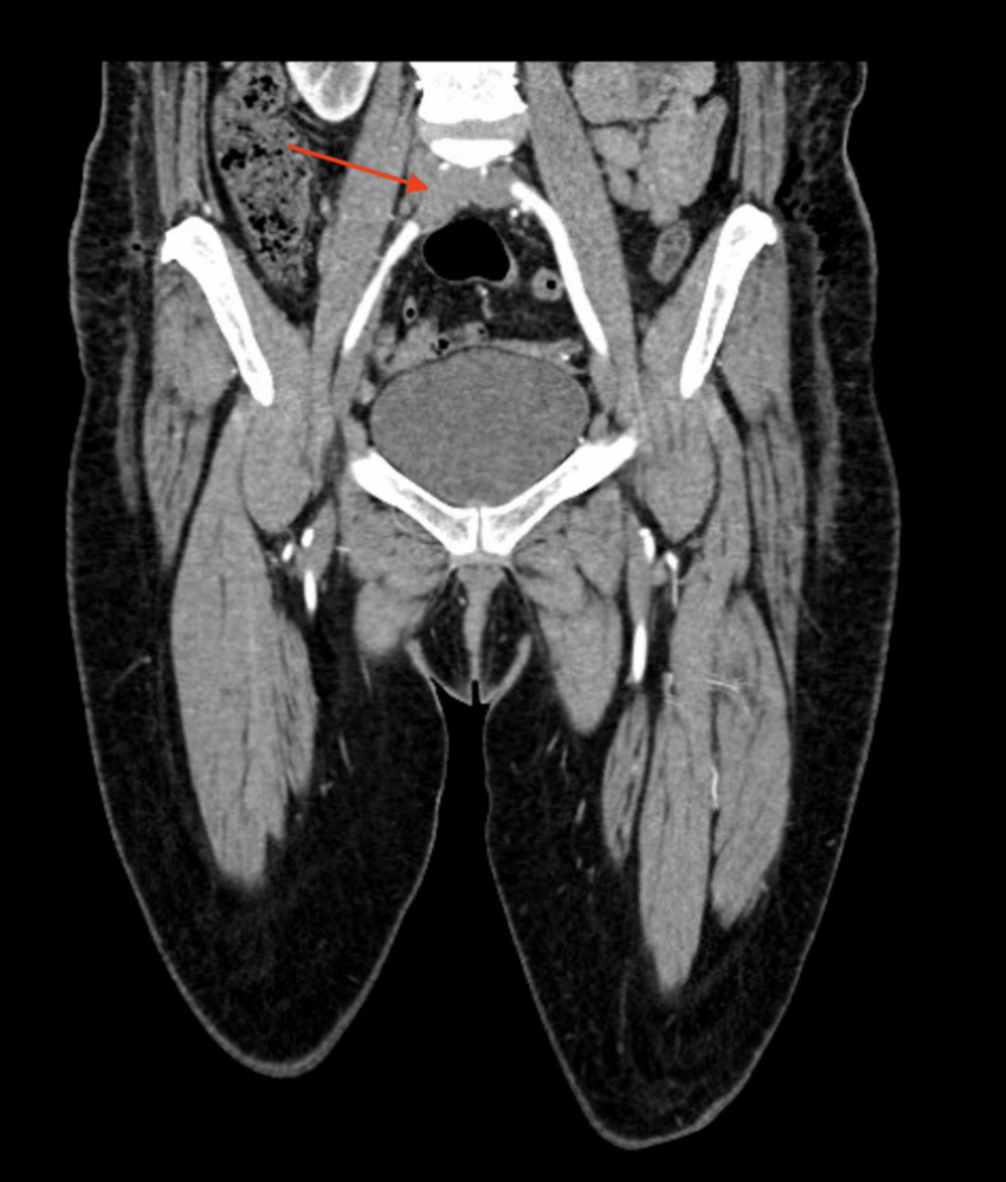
Computed tomography angiogram showing a right common iliac occlusion (arrow)
